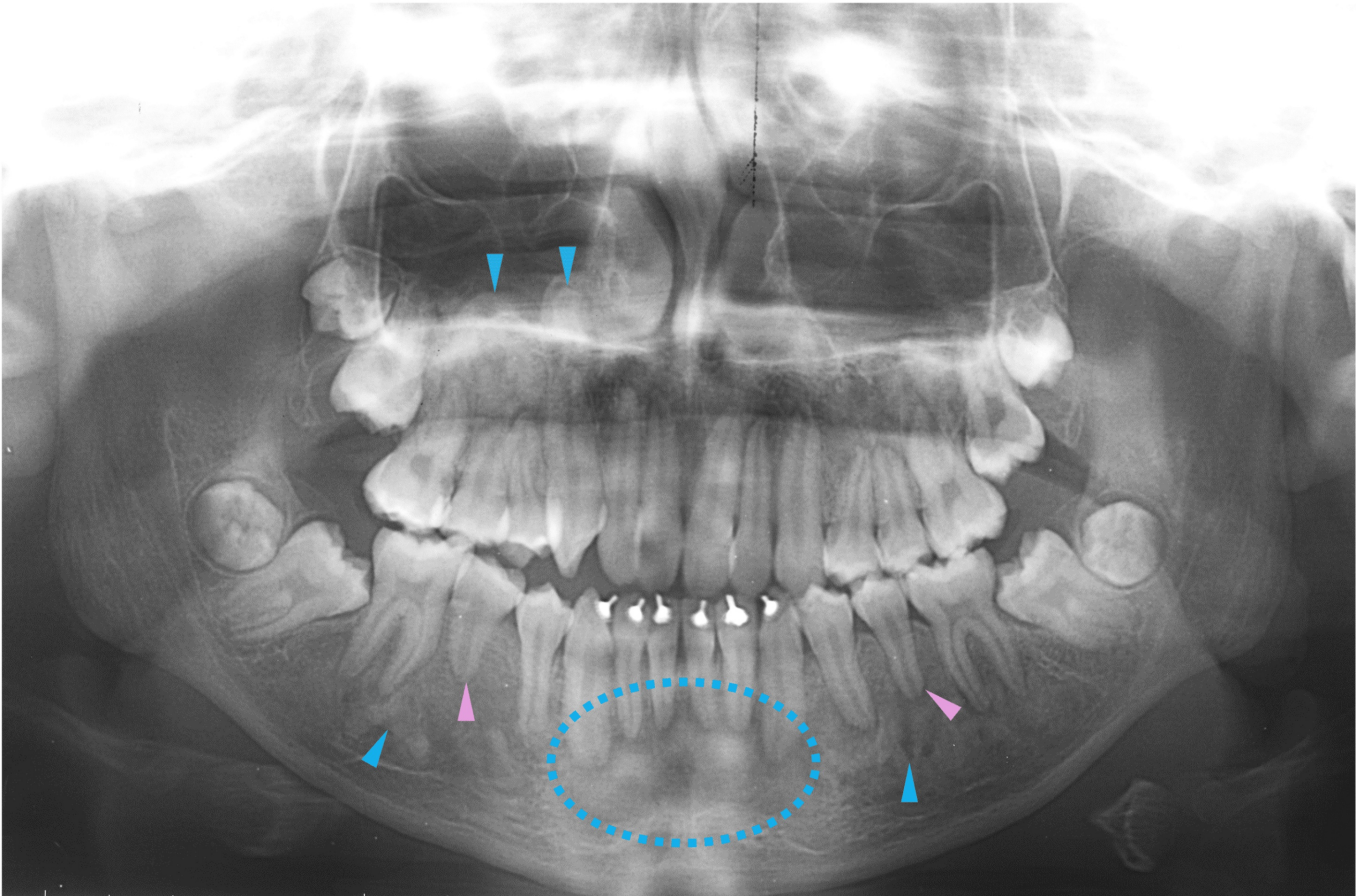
Maxillofacial radiograph at age 13 Blue arrowheads indicate the opacities in the maxillary sinus and new sclerotic lesions at the apical sites of the mandibular premolars. The blue dotted circle indicates the sclerotic lesion in the anterior mandibular region. Purple arrowheads indicate the short roots of the second molars
